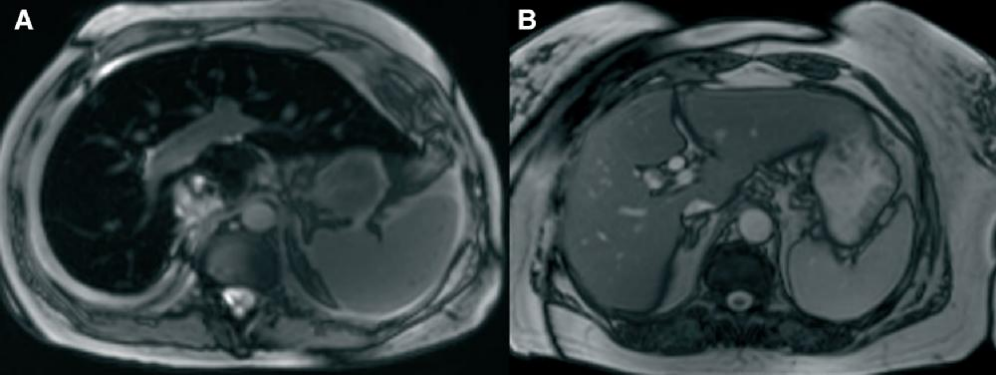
Abdominal magnetic resonance imaging: (*A*) liver with diffusely very low signal intensity; (*B*) liver with normal signal intensity.
